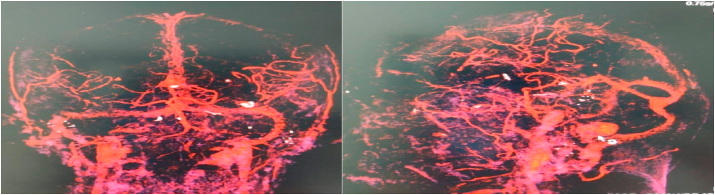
CT Angiography showed no vascular injury or vascular near to the foreign body.
